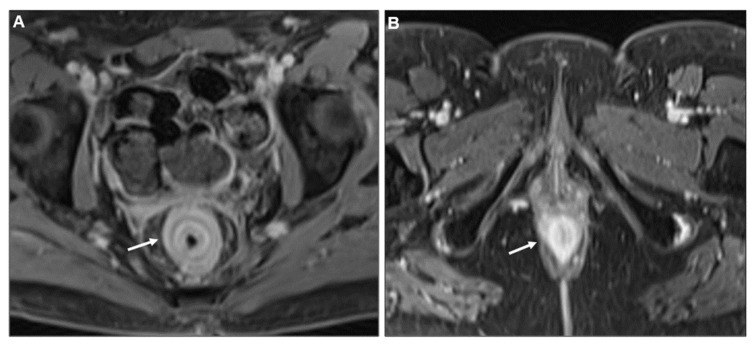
(A,B) MR images: Axial CE-GRE-T1WI. Linitis plastica-like metastasis to the rectum (arrow in (A)) from breast adenocarcinoma with extension to the anal verge (arrow in (B)), likely related to longitudinal intramural spread facilitated by peristaltic and antiperistaltic movements.
